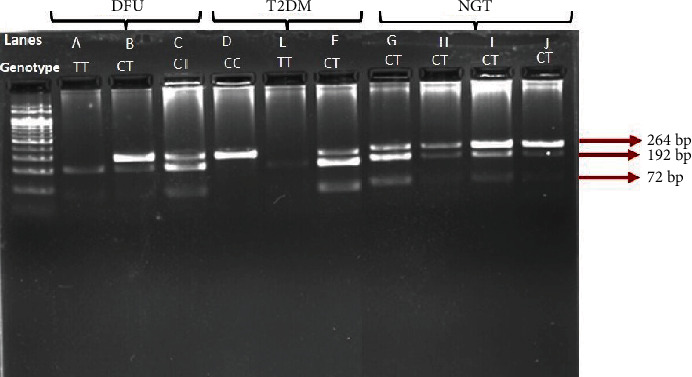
PCR-RFLP results of rs35652124. First lane shows 100 bp DNA ladder. Lanes A and E indicate the “TT” genotype with bands at 192 and 72 bp. Lane D represents CC genotype with a single band at 264 bp alone. Lanes B, C, F, G, H, I, and J indicate CT genotype with three bands at 264, 192, and 72 bps.
